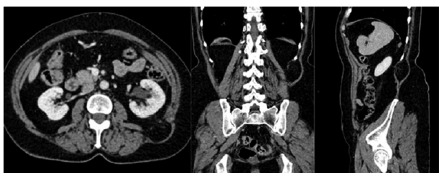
Computed tomography of abdomen revealed protrusion of retroperitoenal fat through the muscles in the upper lumbar triangle topography, defect of about 18 mm (major axis); hernial sac of about 54 mm which was located between the lateral border of the erector muscle of the spine and the medial margin of the internal oblique muscle under the latissimus dorsi muscle, characteristic finding ofGrynfelthernia
